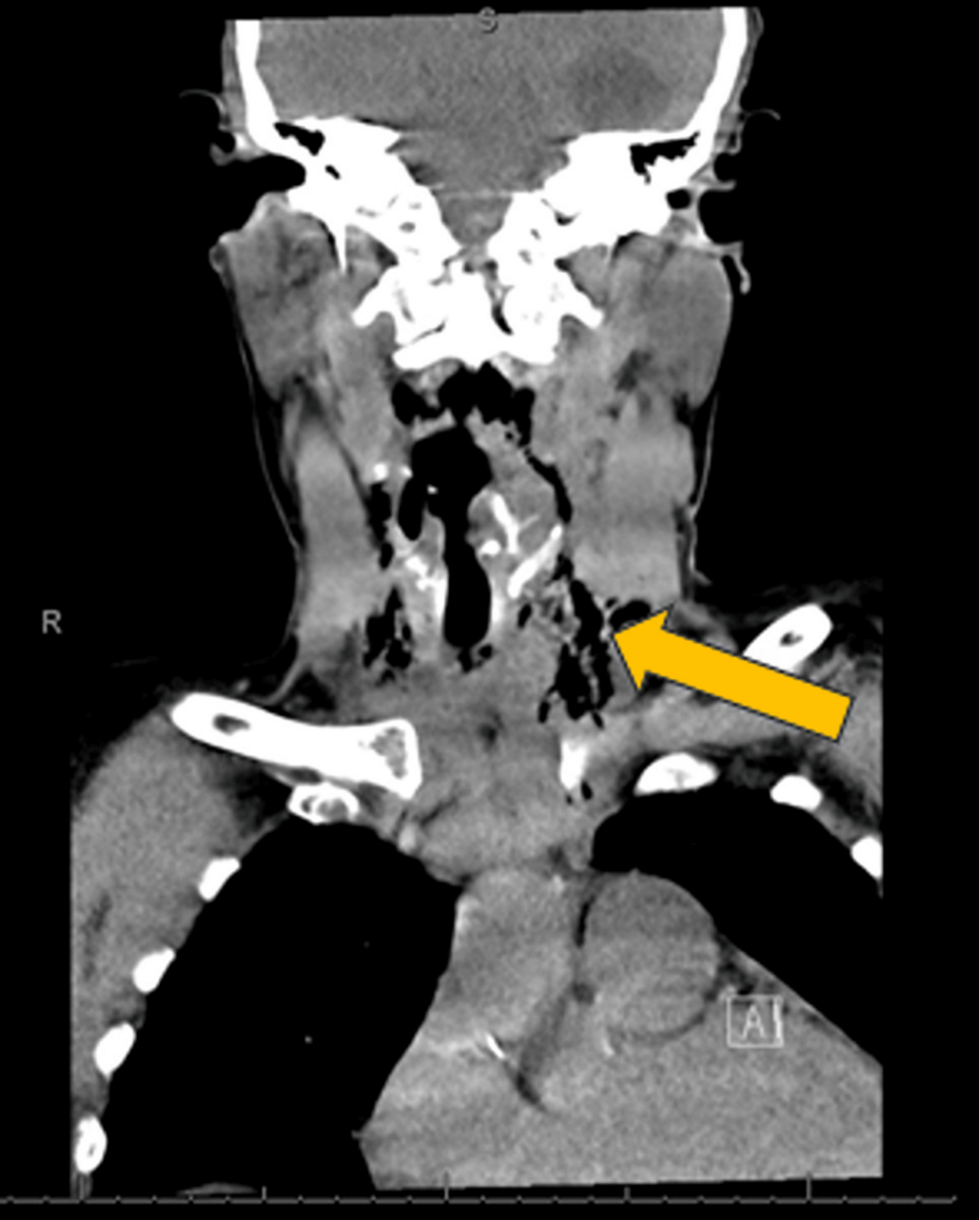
Computed tomography (CT) neck soft tissue Soft tissue air within soft tissue of neck
